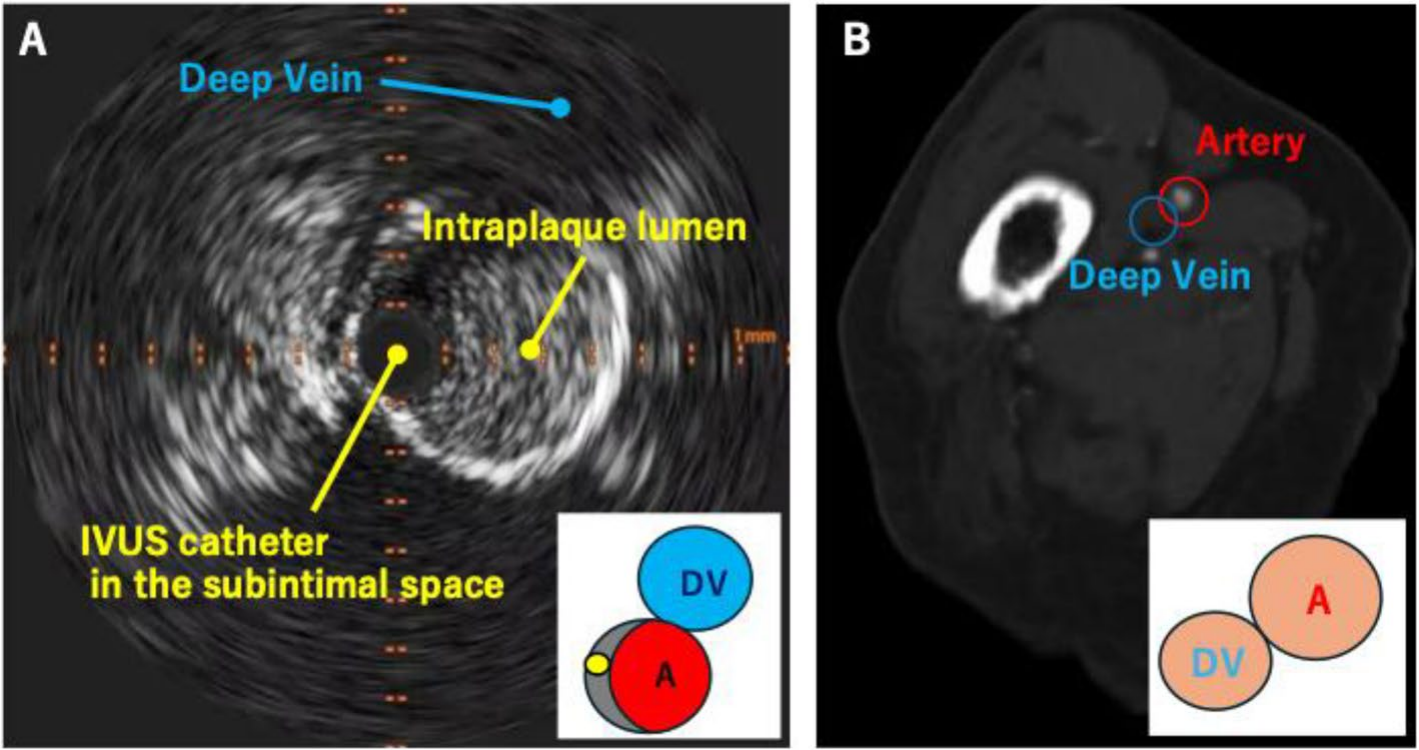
(A) IVUS image; The deep vein is located at the 1 o’clock position relative to the artery on the clock-face orientation. (B) CT image; The deep vein is located at the 8 o’clock position relative to the artery on the clock-face orientation. Abbreviations: DV, deep vein; A, artery
